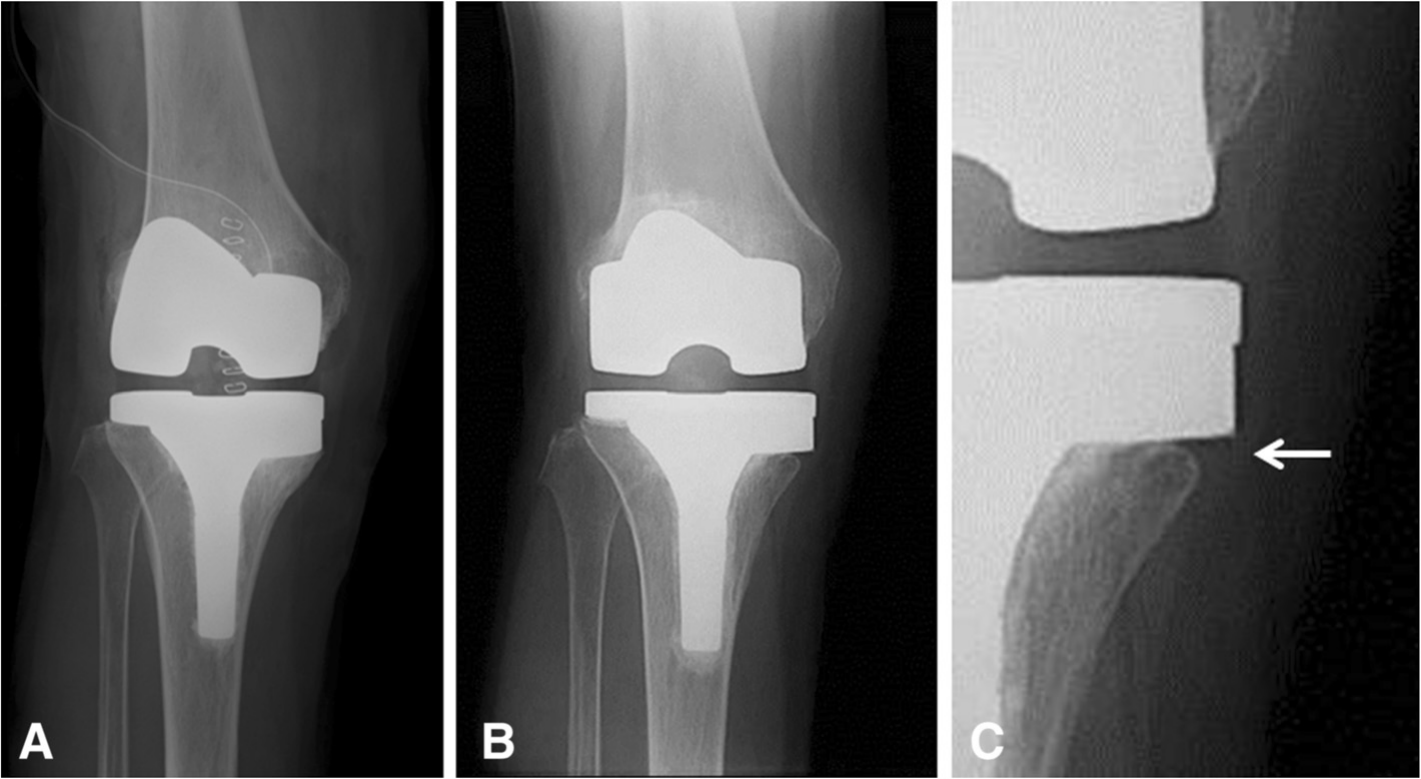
Metal block augmentation was performed for a peripheral defect of the medial tibia in a 72-year-old woman. (A) Immediate postoperative radiograph. (B, C) Radiographs recorded 5 years after surgery with rounding at the medial edge of the tibia (arrow).
